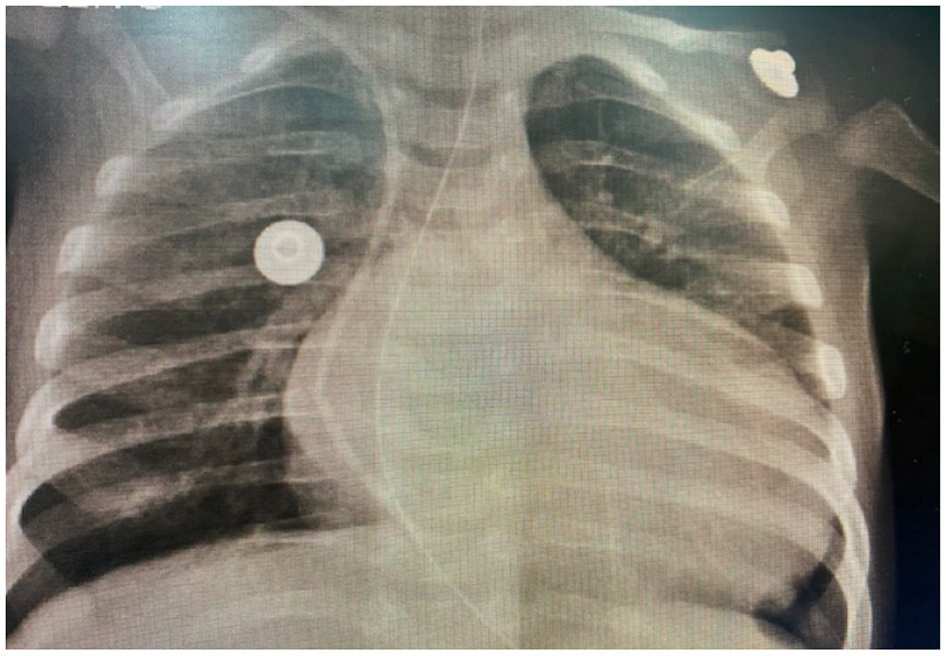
Chest X-ray of the patient, taken on the February 12, 2021.
